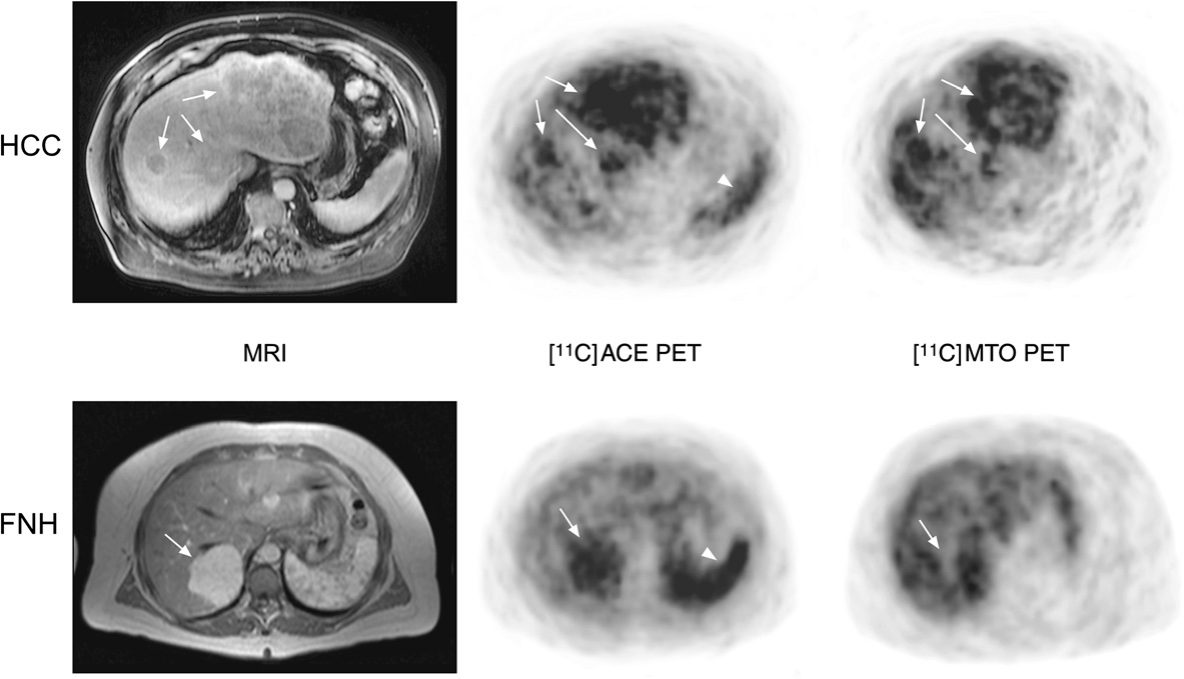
Representative transaxial MRI and PET images of patients with hepatocellular carcinoma and focal nodular hyperplasia. Arrows indicate tumors, and arrowheads indicate normal uptake in the spleen. HCC: A 71-year-old male had three tumors; the largest tumor (diameter, 3 cm) was biopsy-proven as a HCC grade II lesion. FNH: A 43-year-old female had biopsy-proven FNH.
